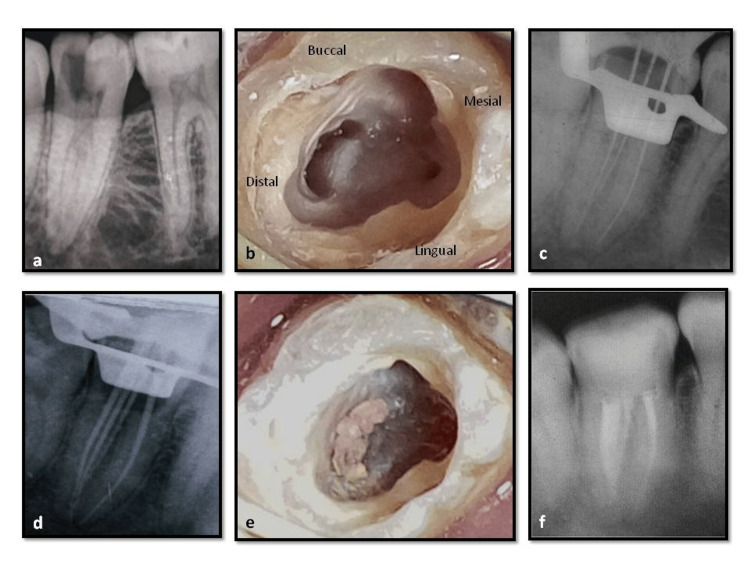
Case report-2 a) Preoperative radiograph, fused mesial and distal roots; b) access opening, mesiobuccal canal orifice with a groove extending continuously to the distal canal with a distinct mesioligual canal, typical semicolon shaped configuration; c) working length radiograph; inserted instruments give the impression of passing through the furcation area; d) master cone radiograph, mesiobuccal and distal canals coverging in the apical third and a separate mesiolingual canal; e) postobturation clinical photograph; f) postoperative radiograph.
